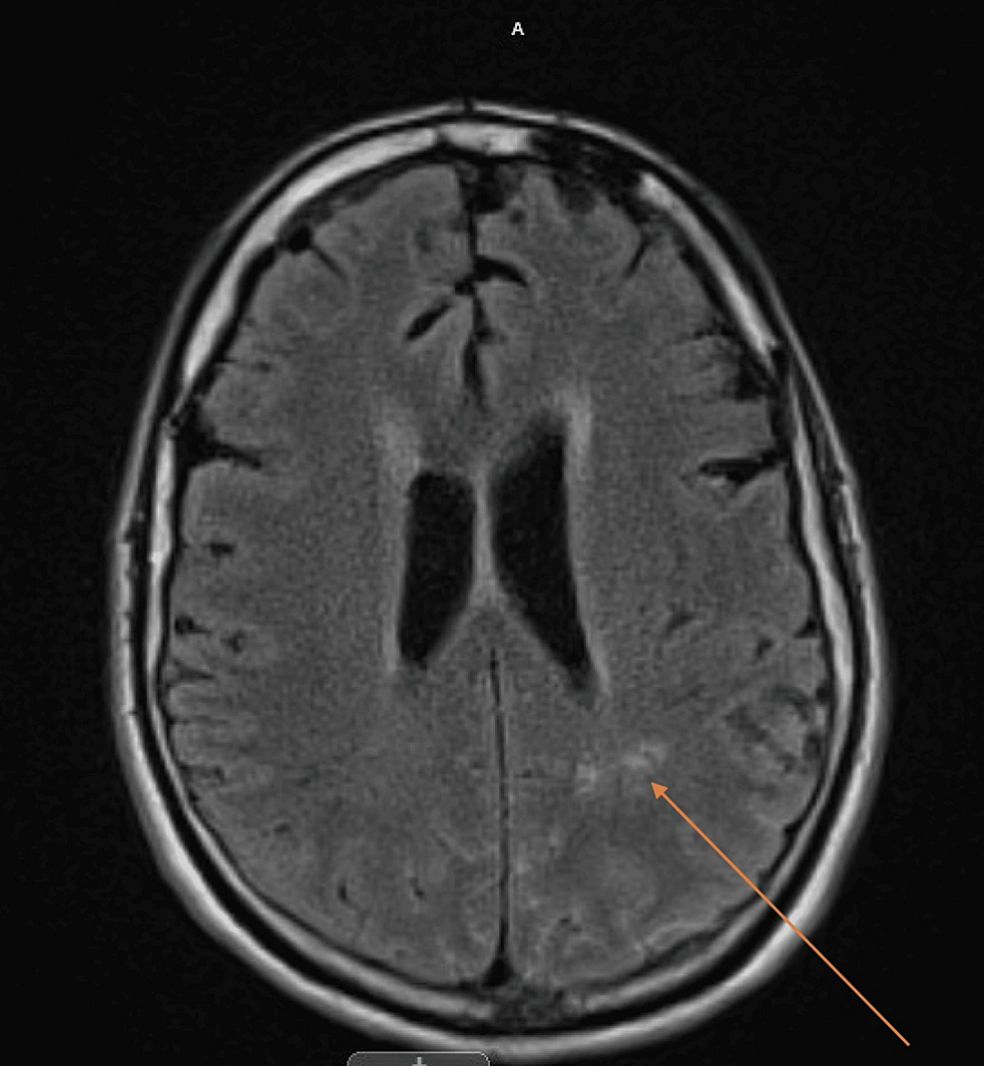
MRI FLAIR window showing subtle meningeal enhancement Meningeal enhancement of the occipital lobe is shown by orange arrow. MRI, magnetic resonance imaging; FLAIR, fluid-attenuated inversion recovery.
